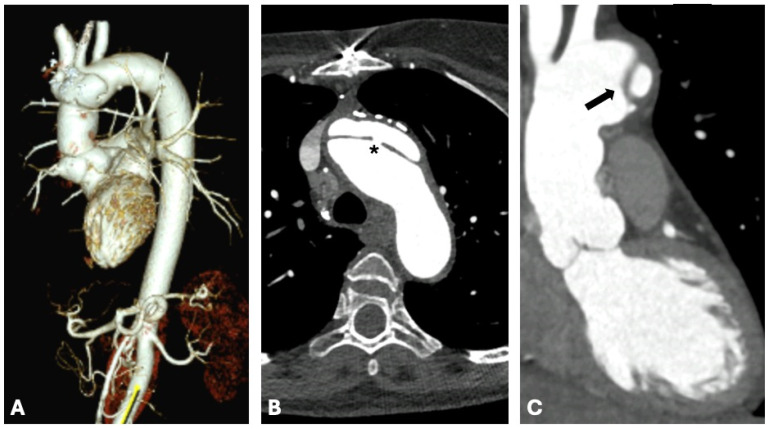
A 34-year-old woman with Marfan syndrome who underwent emergency CT examination on suspicion of aortic dissection. The asterisk and arrow panels (B,C) show the breach and the false lumen, respectively. Panel (A) shows the 3D reconstruction in volume rendering.
